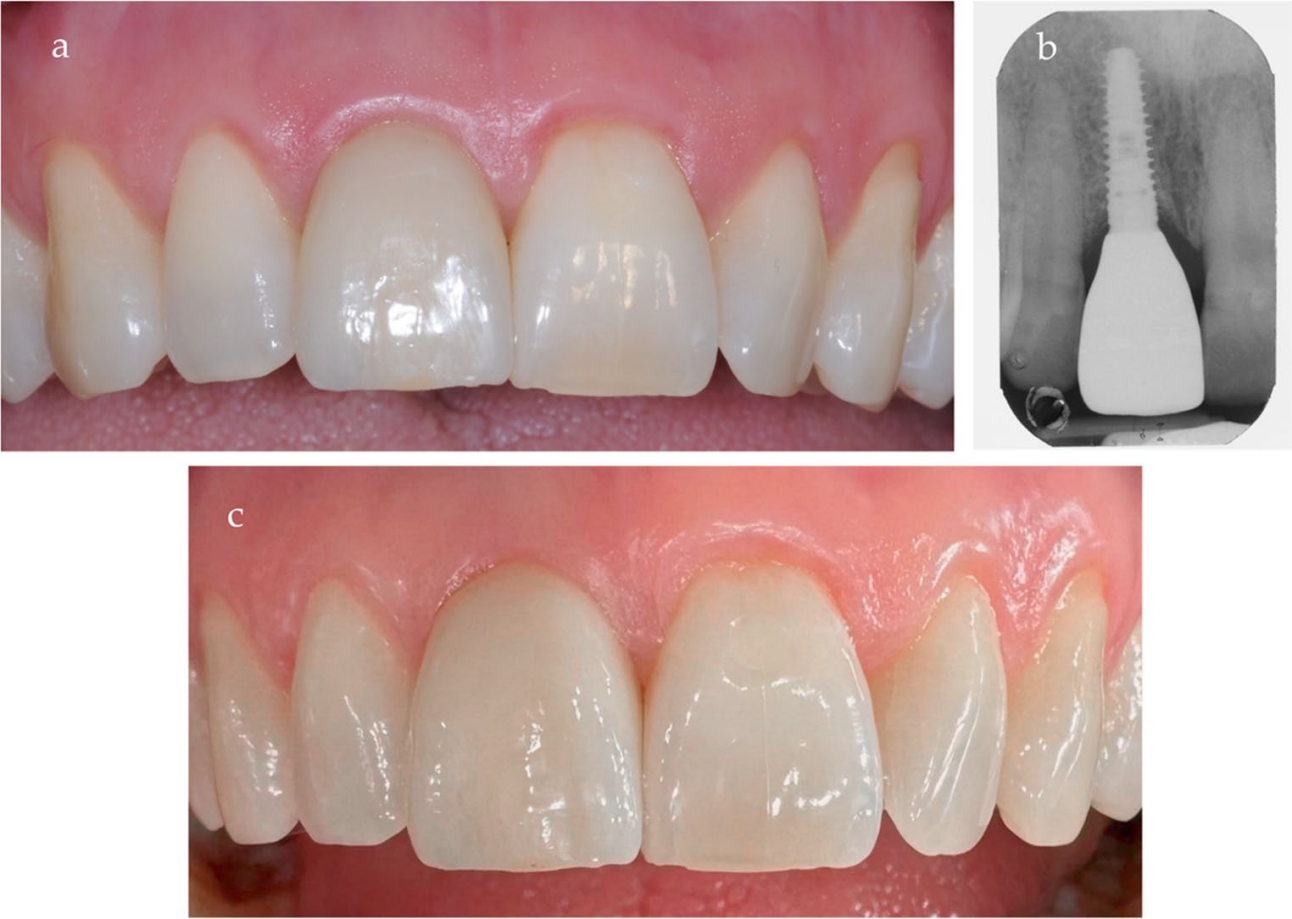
a Final restoration. b Final restoration X-Ray. c 1 year follow-up
